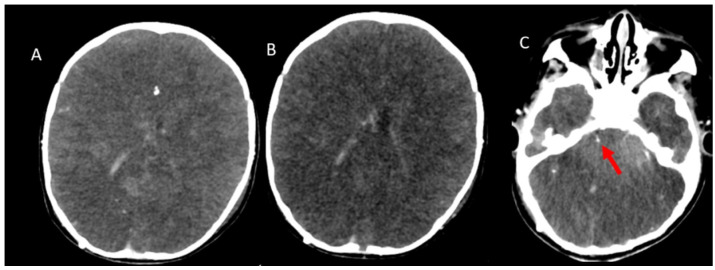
CTA was performed in a 1-year-old boy with clinically confirmed brain death who did not meet the criteria for brain death. (A), Arterial-phase image and (B,C), Venous-phase images reveal an absence of opacification in the branches of the MCAs. Conversely, opacification of the SPV is seen (red arrow). CTA: CT angiography, MCA: Middle cerebral artery, ICV: Internal cerebral vein, SPV: Superior petrosal vein.
